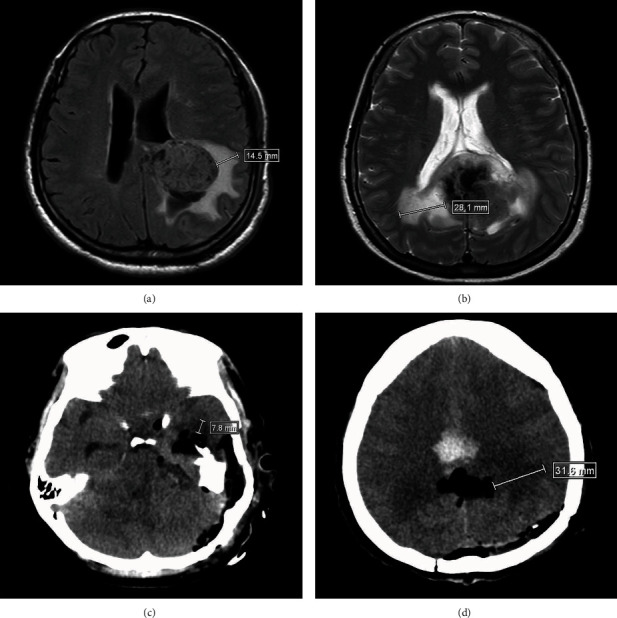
Evaluation of peritumoral brain edema (PTBE) and pericavity brain edema by magnetic resonance imaging (MRI) and computed tomography (CT), respectively. (a) MRI scan showing mild PTBE before surgery. (b) MRI scan showing severe PTBE before surgery. (c) CT scan showing mild brain edema around the cavity after surgery. (d) CT scan showing severe brain edema around the cavity after surgery.
